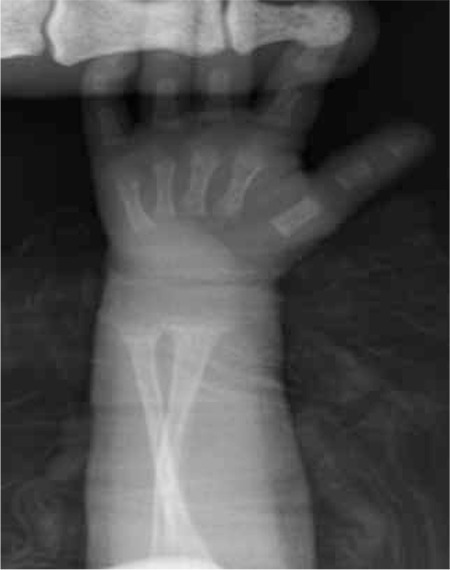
Case 2- wrist X-ray demonstrating metaphyseal widening and irregularity
